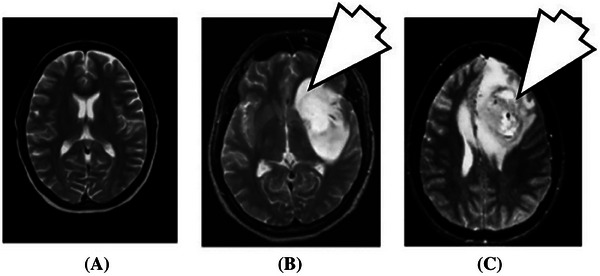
Brain MRI acquisition reporting A) normal brain, B) benign tumor, and C) malignant tumor. Adapted from Tandel et al. (2019).
